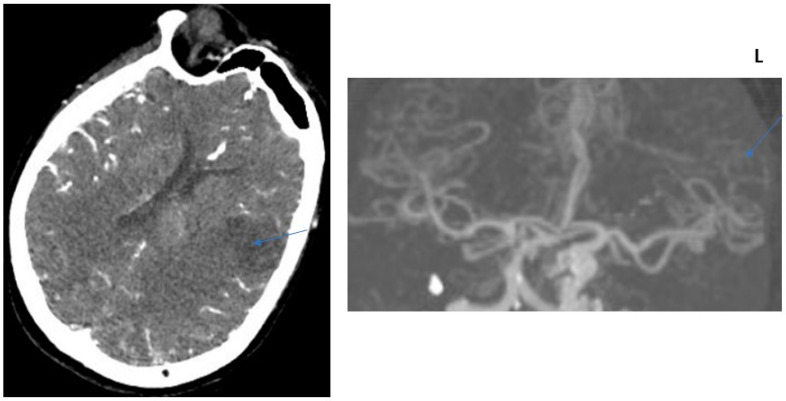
COLLATERAL SCORE: 2–71-year-old female patients. Left MCA infarction. NIHH Score: 18. CT angiography was performed 6 h after the acquisition of clinical findings. Left image, “Subtracted MIP image obtained from arterial phase images”. Right image, “Raw image in the axial plane obtained in the arterial phase after contrast injection”. Arrows represent collateral score area.
